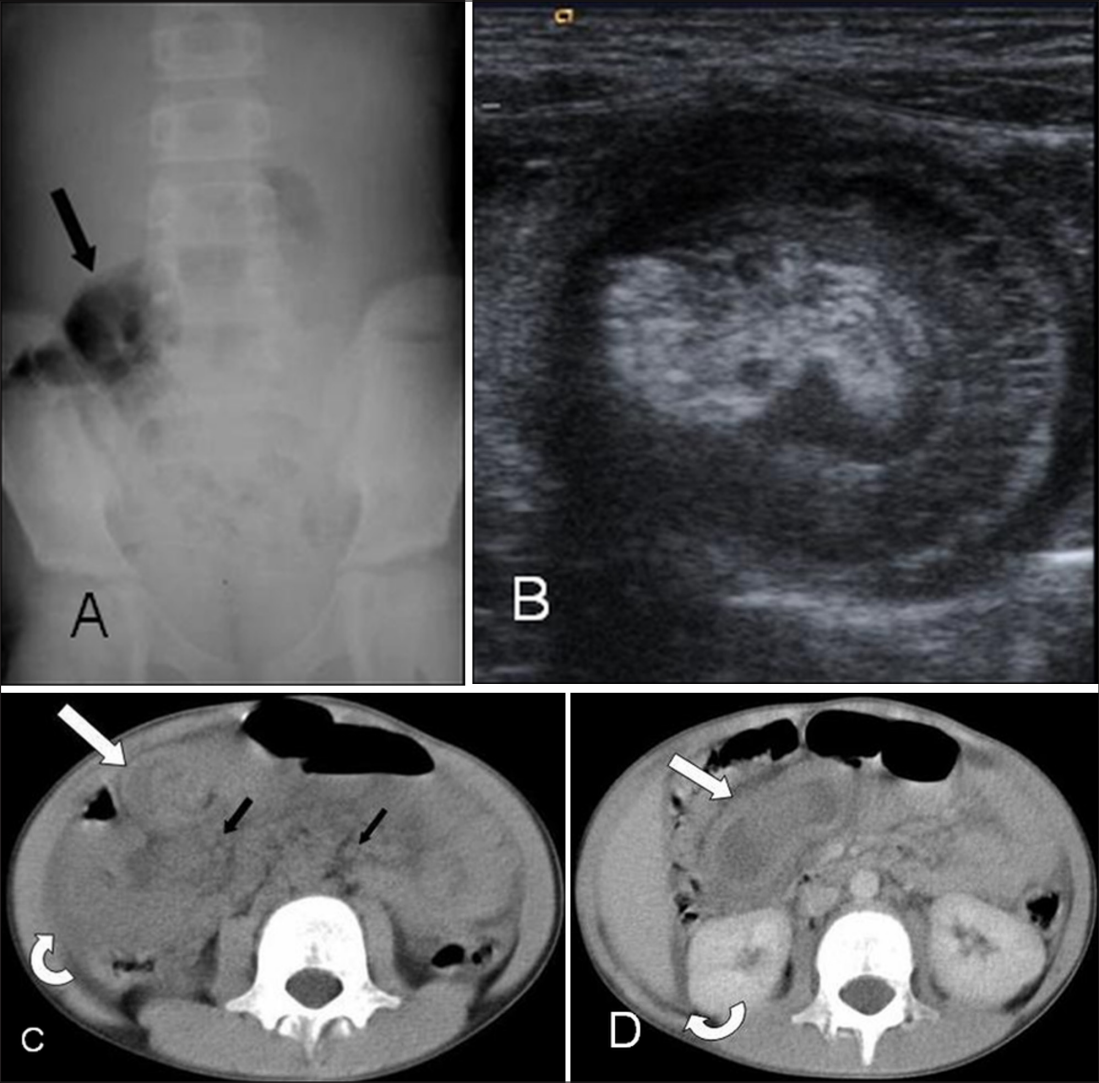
A nine-year-old boy with Henoch-Schoeinlein purpura presenting with abdominal pain, who received the post-operative diagnosis of ileoileal invagination. (A) PAX examination shows no marked radiopathological finding except bowel distention with gas in the right-lower abdominal quadrant, indicated by black arrow. (B) The zoomed ultrasound image shows the target sign which is consistent with intestinal invagination is present. CT without contrast (C) and with contrast (D) show the level of invagination. Invaginated intestinal segments and thickening in intestinal walls—thought to be ileoileal invagination—formed the target sign (white arrow). Furthermore, paraaortic lymph nodes adjacent to the invagination (black arrow) and free fluid around the liver and intestinal loops (curved arrow) were observed.
